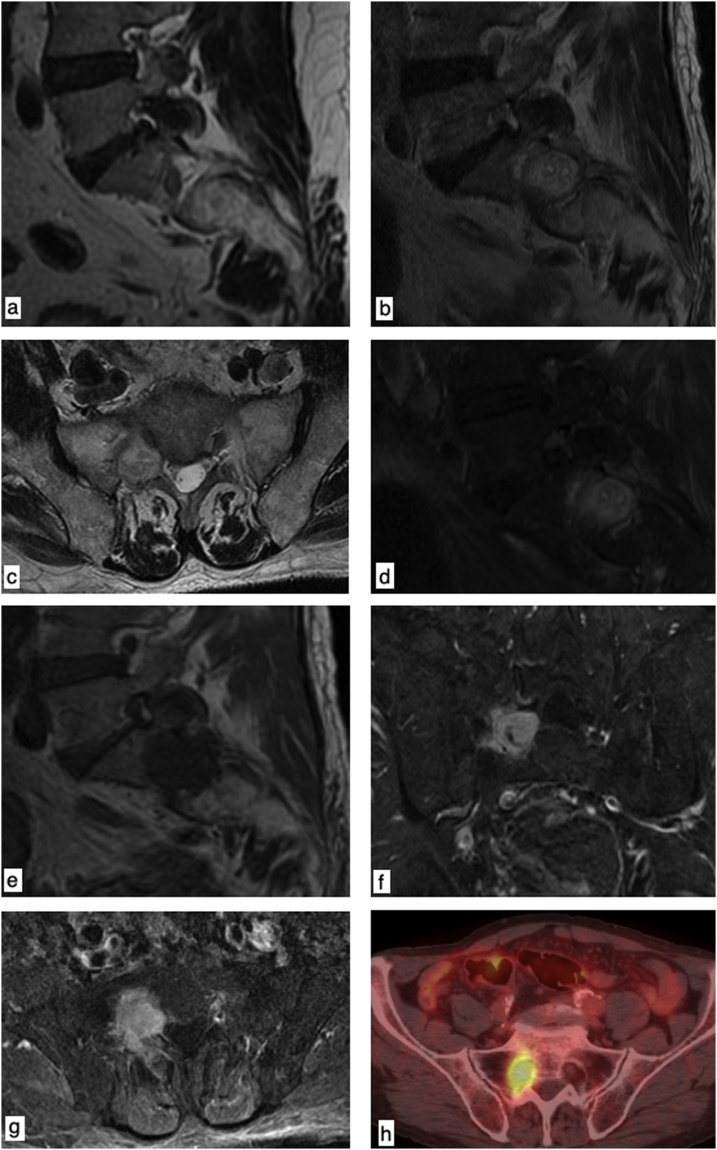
Sagittal TSE T2-weighted image (July 2015) showing no abnormal findings in the L5-S1 foraminal space (a). Sagittal TSE T2-weighted image (b), axial TSE T2-weighted image (c) and sagittal fat-sat TSE T2-weighted image (d) showing a hyperintense round-shaped lesion involving the right L5-S1 foraminal space. Sagittal SE T1-weighted image (e) showing an hypointense mass in the L5-S1 foraminal space; coronal (f) and axial (g) fat-sat SE T1-weighted image after intravenous contrast administration showing avid enhancement of the lesion. 18F – FDG (18F-Fluorodeoxyglucose) CT-PET (Fig. 2h) showing focal hyperaccumulation within the sacral foramina of right S1 nerve root (SUV max. 5.2).
